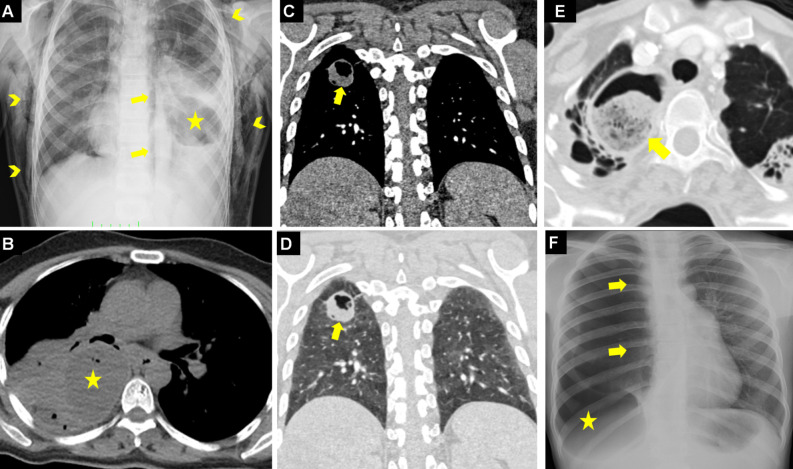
Chest radiograph (A) shows pneumomediastinum (arrows) and diffuse subcutaneous emphysema (arrowheads) developed secondary to a ruptured cyst (asterisk). Axial computed tomography (CT) (B) shows a hydatid cyst (HC) containing air bubbles, requiring differential diagnosis from a slightly high-density abscess. Mediastinal (C) and parenchymal (D) coronal CT of the same patient show the HC (arrows) consistent with a cavitary lesion appearance radiologically developed after the evacuation of cyst content. Computed tomography (E) shows debris (arrow) within a chronic and complicated cyst cavity mimicking an aspergilloma lesion. Chest radiograph (F) shows a life-threatening tension pneumothorax on the right caused by cyst rupture (asterisk), pushing the mediastinum and heart to the opposite side (arrows).
